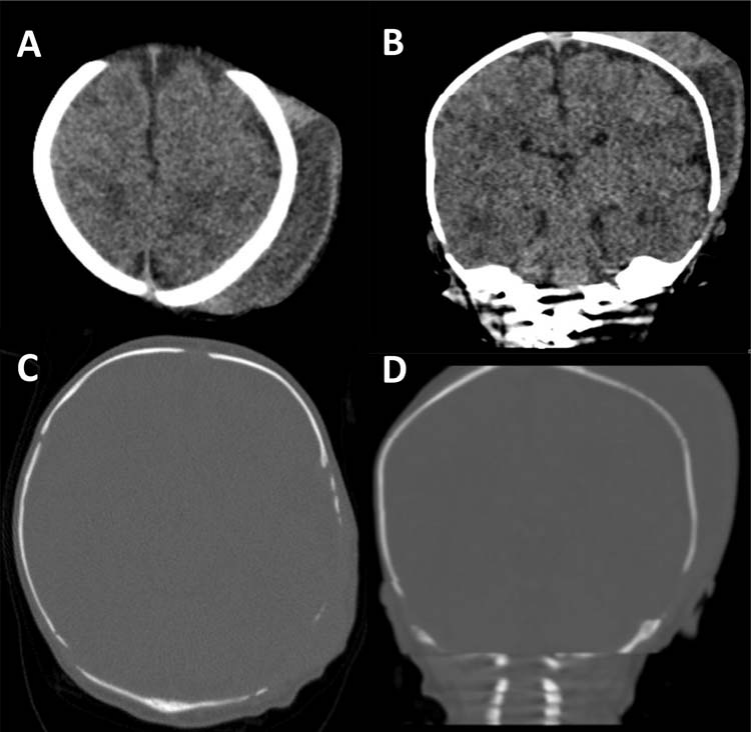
CT scan of our patient. In (A) and (B) the size of the cephalhaematoma can clearly be seen. In (C) and (D) the thinned, moth-eaten appearance of the left parietal region is well demonstrated.
